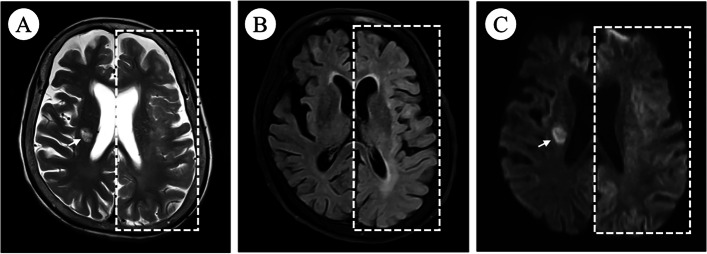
Repeat brain MRI after twenty-eight hours. Repeat brain MRI showed mild gyral hyperintense on axial T2WI (A), FLAIR (B), and DWI (C) in the left brain (dotted square); subacute cerebral infarction near the right lateral ventricle (white arrow with tail)
